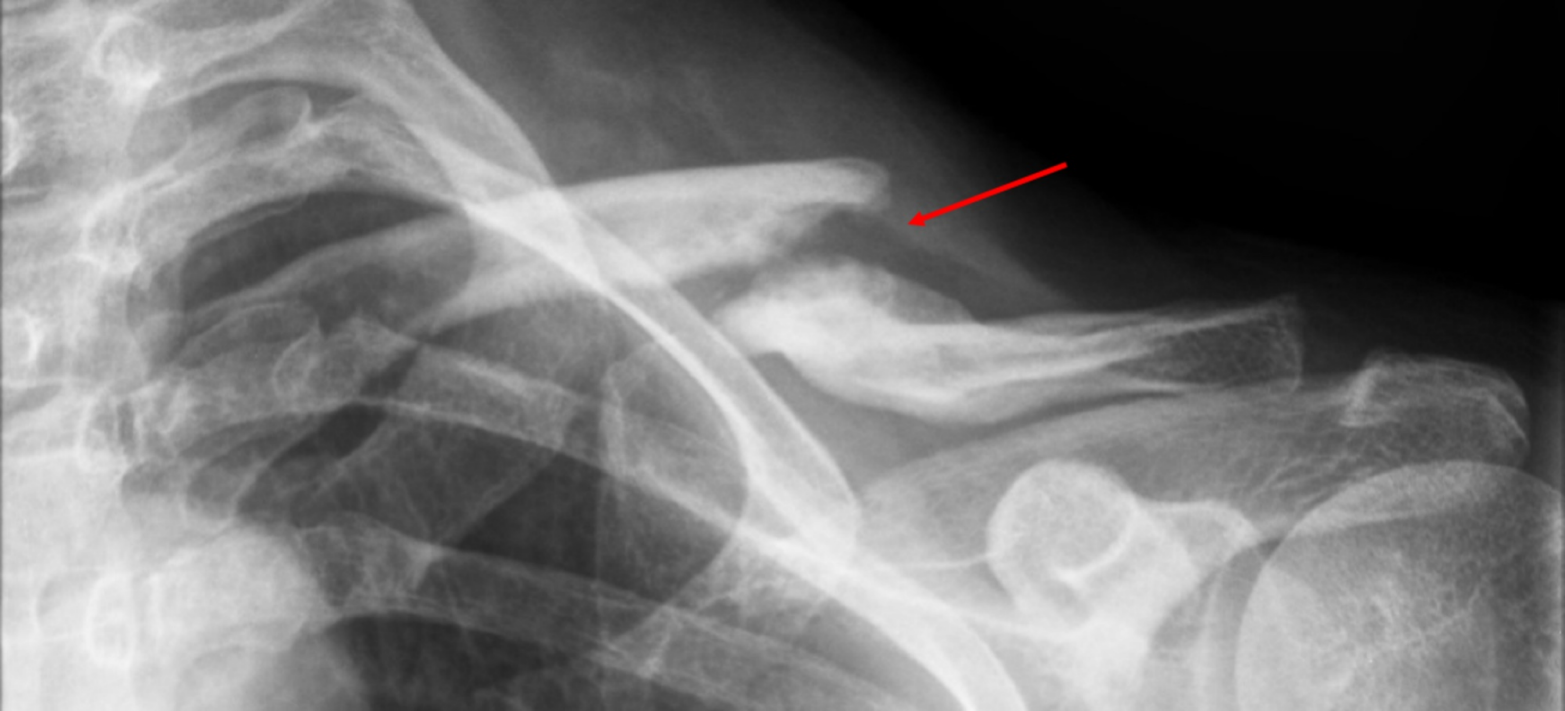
X-Ray example of a midshaft clavicle fracture non-union Red arrow: non-union
